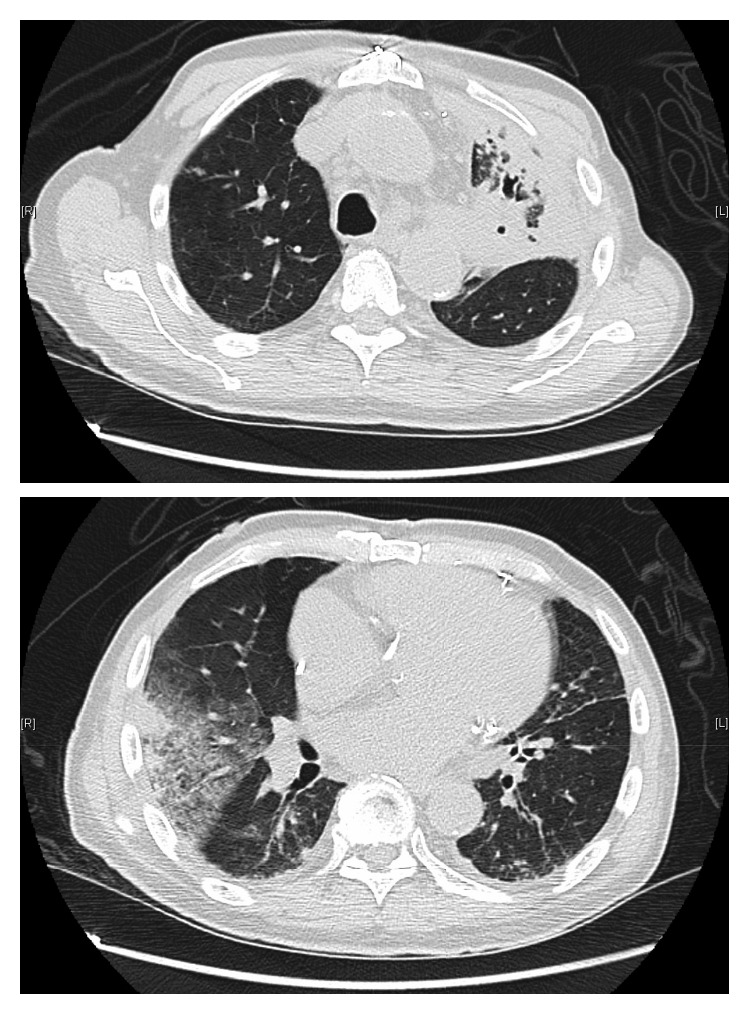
CT chest scan on admission: bronchiectasis and consolidation in the left upper lobe in addition to peripheral consolidation in the right upper lobe with adjacent ground-glass opacification.
